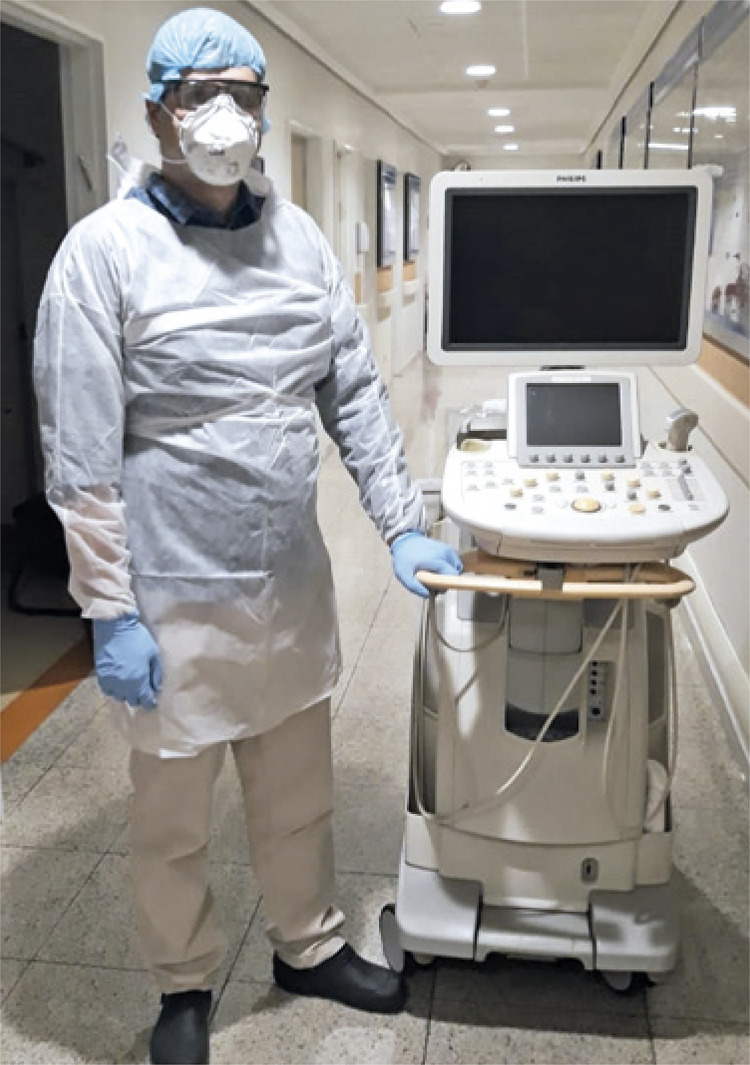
Radiologists perform all the ultrasound examinations in our institution, and were instructed to carried out exams of the COVID-19 patients at bedside. Personal protective equipment, such as gloves, gowns, face mask, and safety glasses, with the respective training of usage was made available to all involved
